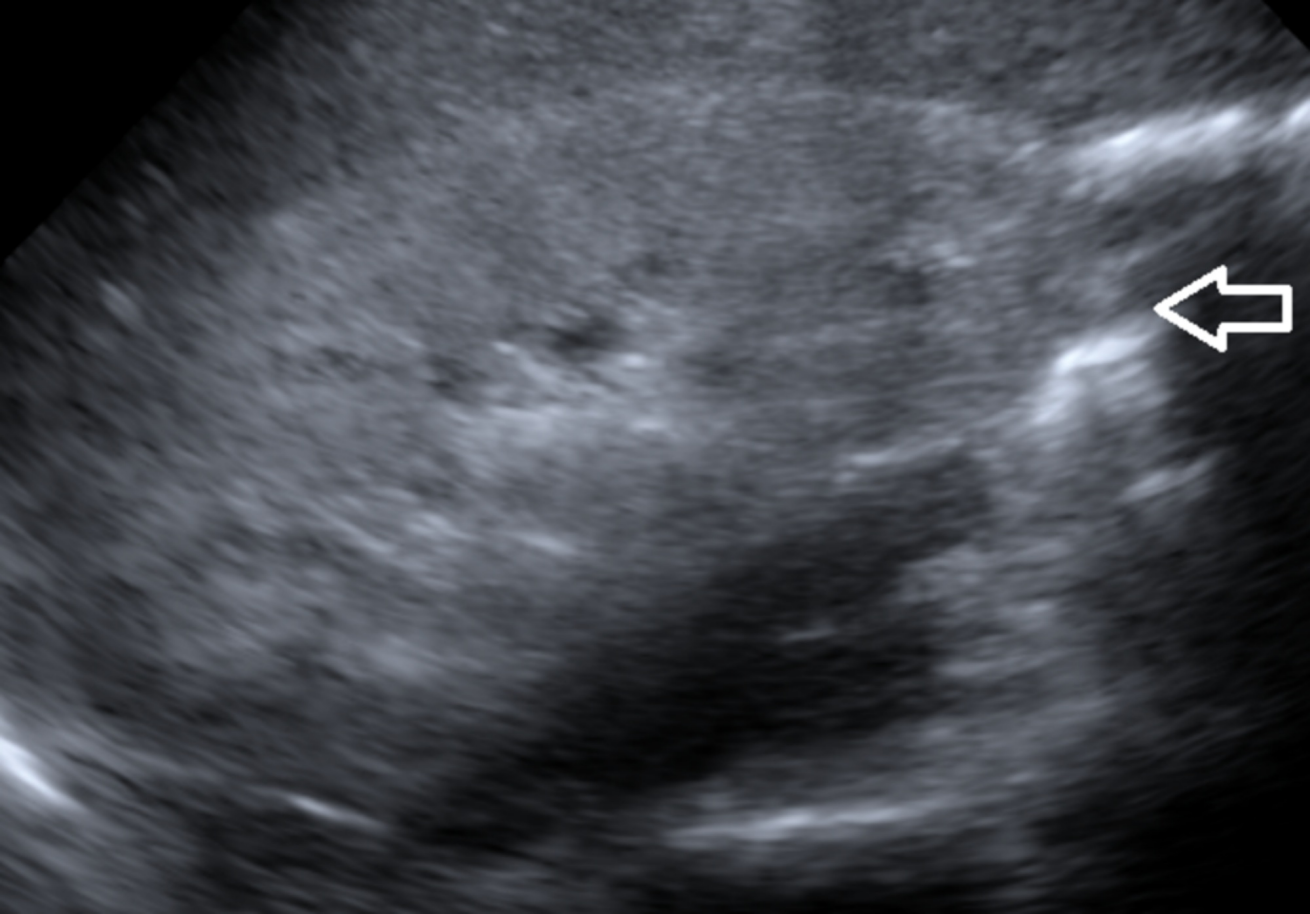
Ultrasound right kidney White arrow points towards increased echogenicity in renal cortex.
